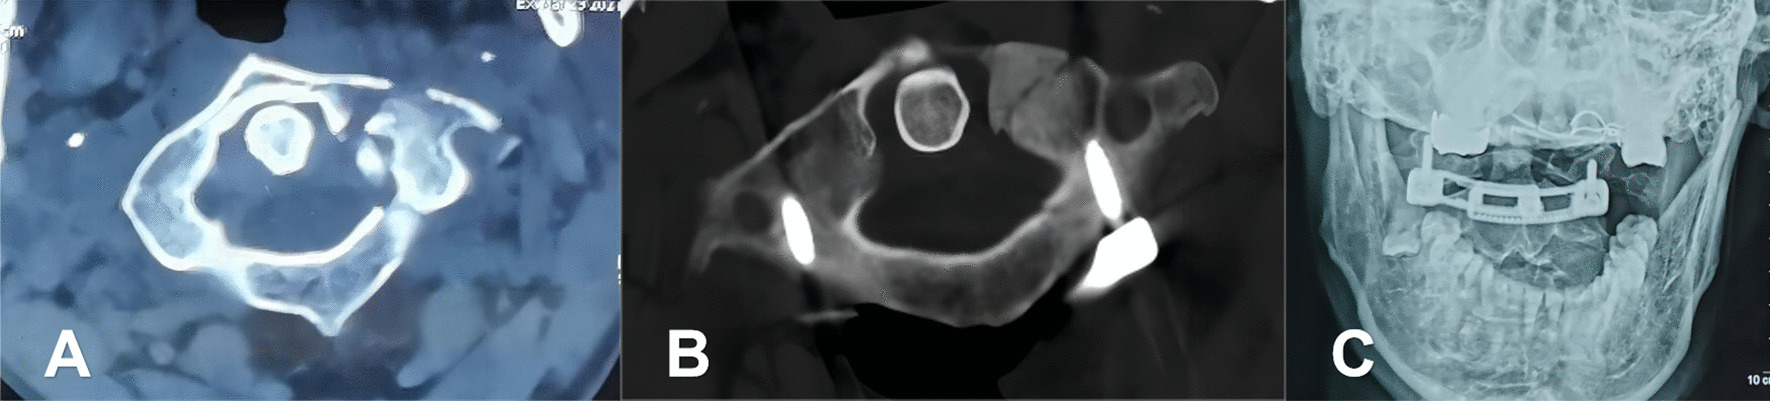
A A 55-year-old male presented with neck pain from a heavy object injury and a fracture of the anterior atlas arch on CT examination; B postoperative CT scans revealed satisfactory reduction; C postoperative open mouth X-ray image
